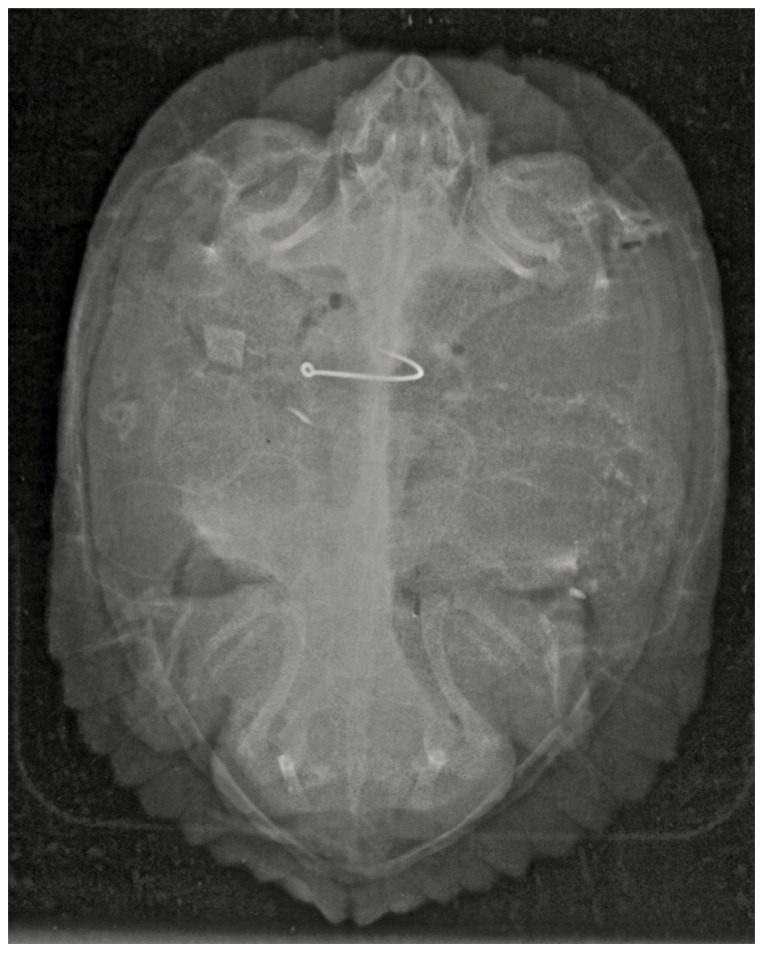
X-ray of a gravid Pond Slider (*Trachemys scripta*) captured in Tennessee containing a fish hook. Image has been enhanced to improve hook visibility.
